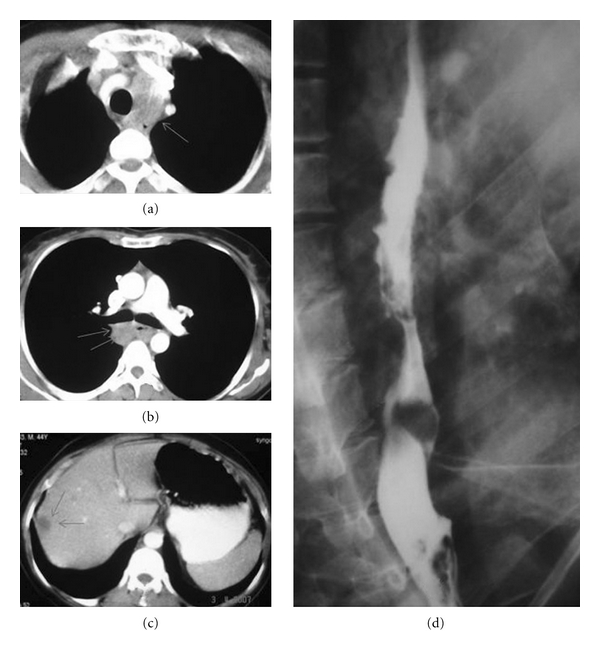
(a, b, c) Thorax CT revealed the multiple mediastinal lymph nodes up to 2-3 cm and hepatic metastasis (arrows). (d) The barium-contrasted esophageal graphy is showing mucosal irregularity.
